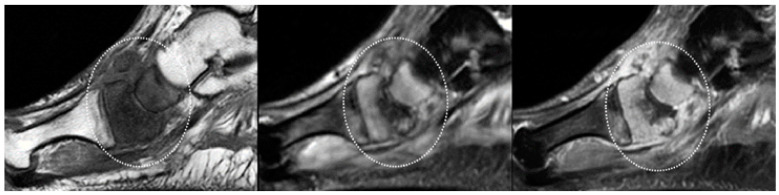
OM of the scaphoid and medial cuneiform. (From left to right) Sagittal T1-weighted, T2-fat-suppressed, and post-contrast T1-weighted fat-suppressed MRI clearly show that the marrow in the scaphoid and medial cuneiform has a low signal on a T1-weighted image (circle in left panel), increased signal on a fluid-sensitive fat-suppressed image (circle in middle panel), and post-gadolinium enhancement (circle in panel). These findings are indicative of OM.
